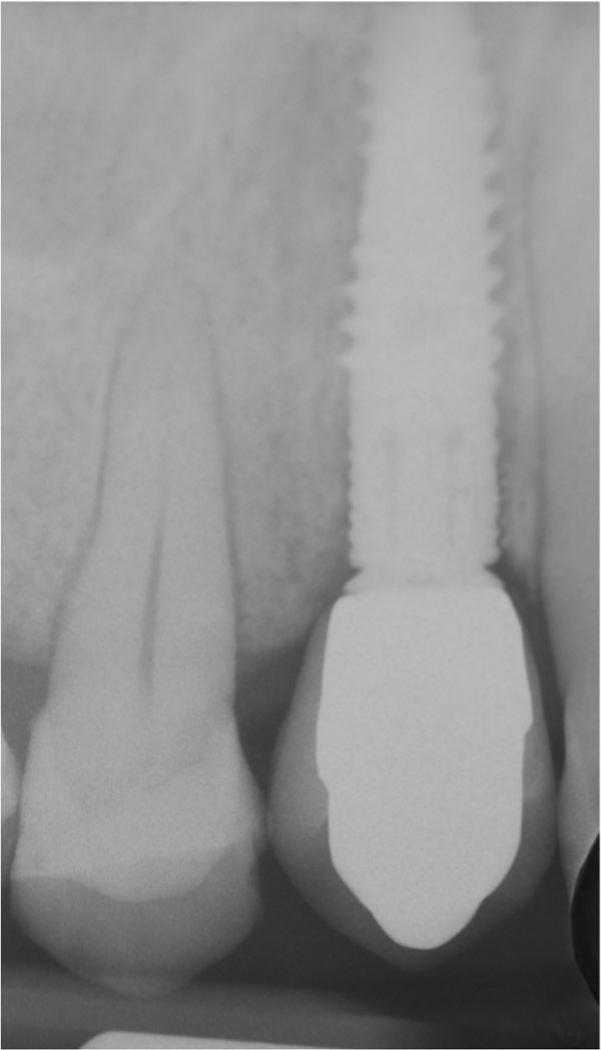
Radiographic evaluation of the postextractive implant at 6 months
